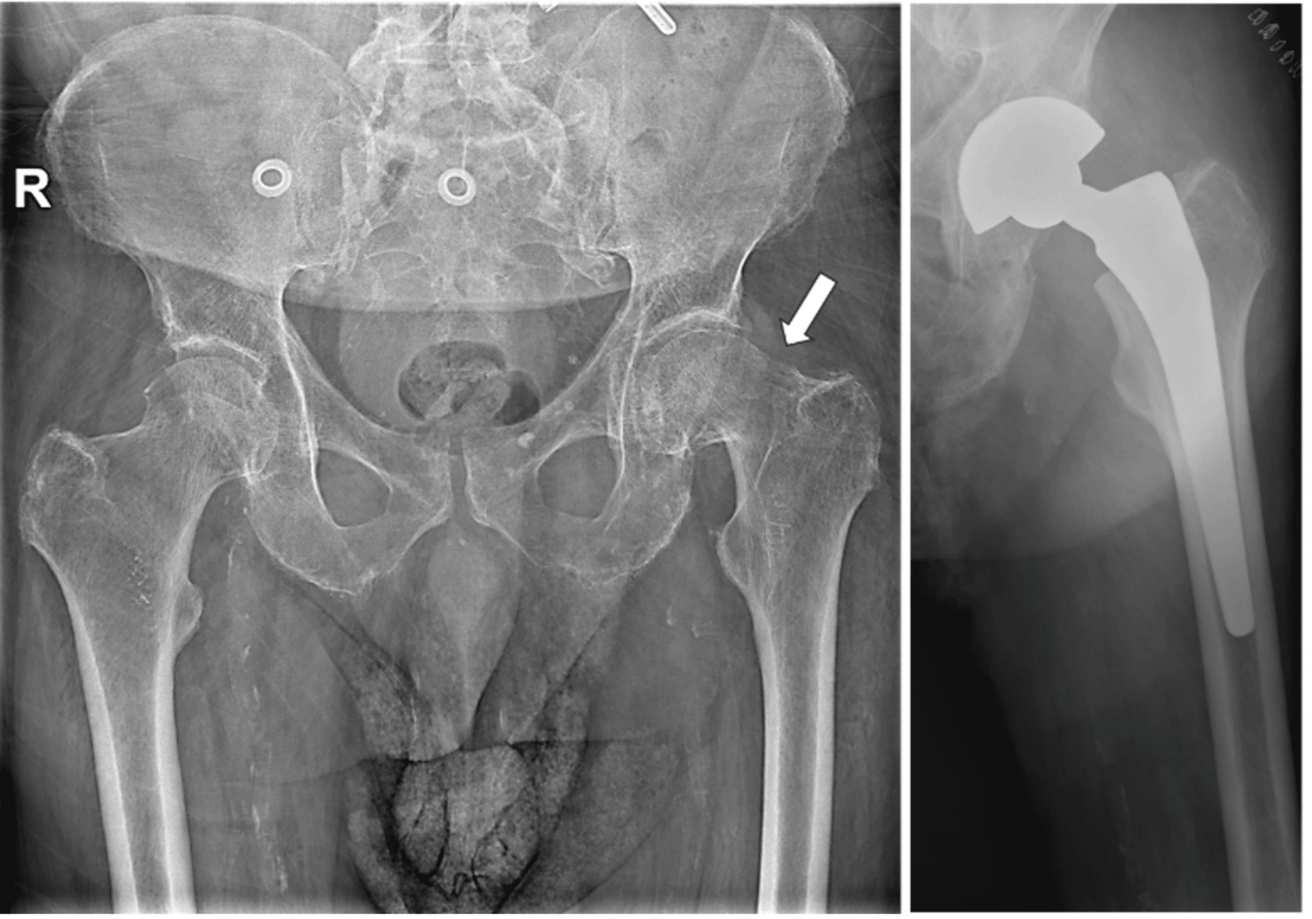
Pelvic radiograph showing displaced femoral neck fracture (arrow) treated with dual‑mobility total hip arthroplasty.
